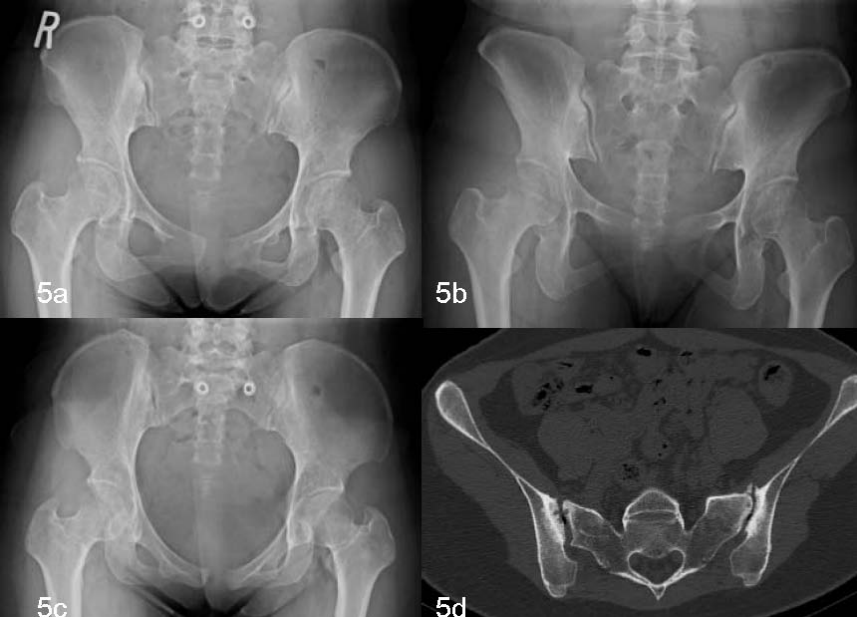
X-rays showing pelvic diastasis disruption reduced to fifteen millimeters. CT showing the right sacroiliac joint was not widened but associated with sclerosis consistent with sacroiliitis.
